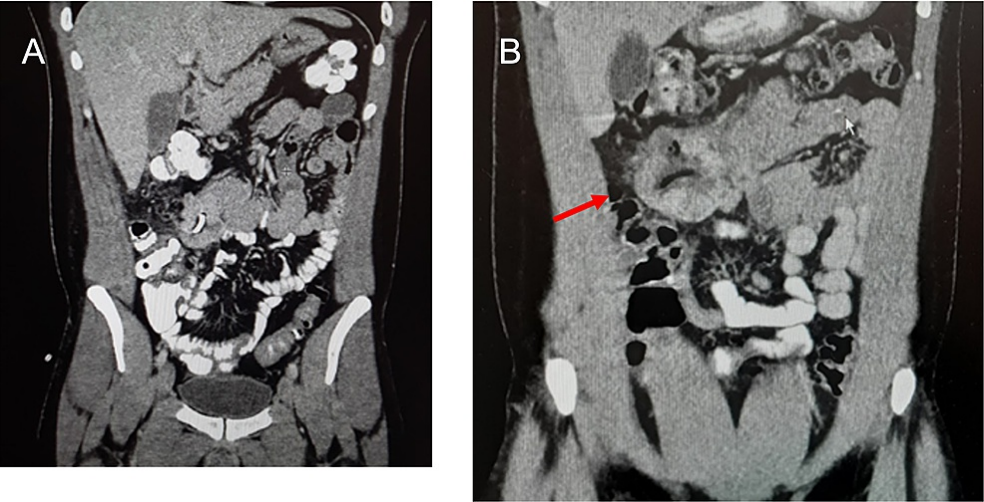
Side-by-side comparison of CT abdomen and pelvis three weeks apart. (A) Coronal CT scan after percutaneous drainage of the abscess was performed. (B) Coronal CT of the same patient three weeks later shows an abscess with complex features suggesting a mass of the small bowel. The size of the mass is 6 x 5.5 x 5 cm, similar to the abscess drained during the initial presentation.
